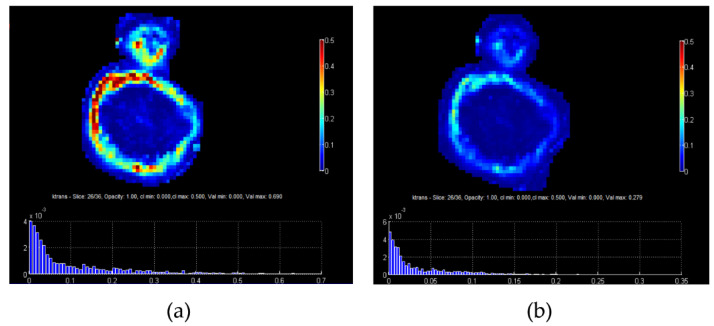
(a) ktrans map of a tumor from PK analysis using AIF measured directly from the MR image, while for the conversion from signal to CA concentration the multiple flip angles method (mFAs) was used, (b) ktrans map of the same tumor using a population based AIF from Fritz and Hansen.
